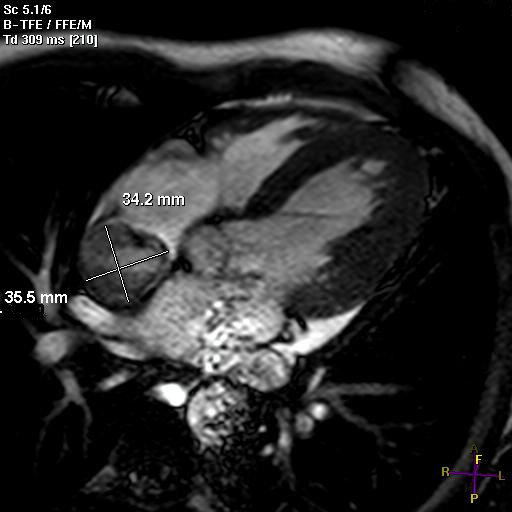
Cardiac magnetic resonance imaging demonstrating a mass in the right atrium involving the left atrial dome and atrial septum.
